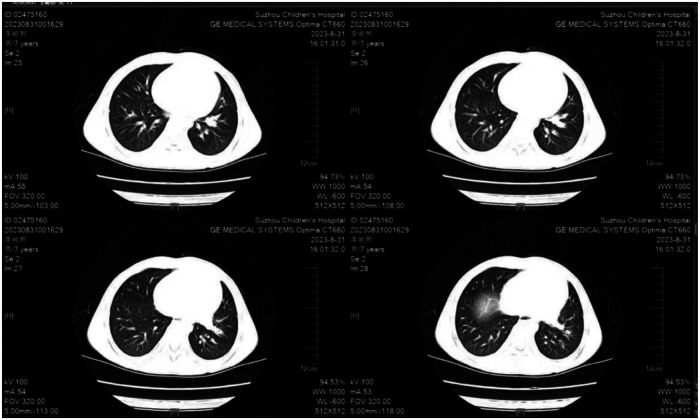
Chest CT (2023-8-31): increased bronchovascular markings in both lungs, with a few patchy opacities in the left lower lobe.
